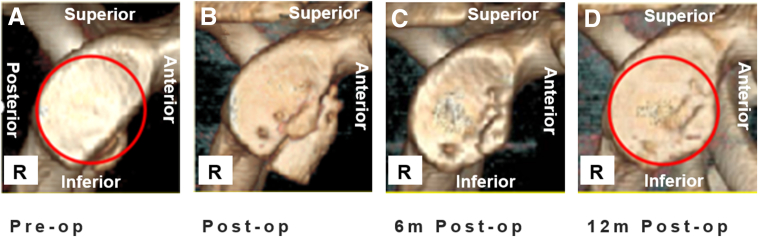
Three-dimensional computed tomography en face views of the right glenoid. (A) Preoperative image. (B) Postoperative image. (C) Image at 6 months, showing complete bone healing and partial remodeling. (D) Image at 12 months, showing complete bone healing and remodeling.
